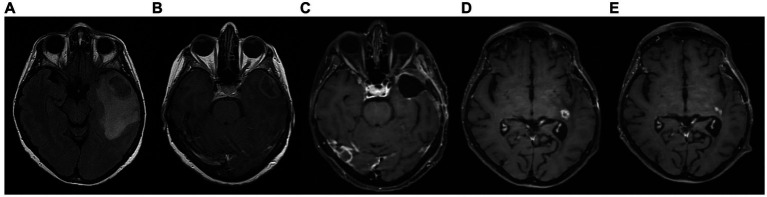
Patient 3: (A) brain MRI on diagnosis (T2/FLAIR) (B) brain MRI on diagnosis (T1 with contrast) (C) 9-month follow up brain MRI (T1 with contrast) (D) GBM relapse, 12-month follow up brain MRI (T1 with contrast) (E) 30-month follow up brain MRI (T1 with contrast).
